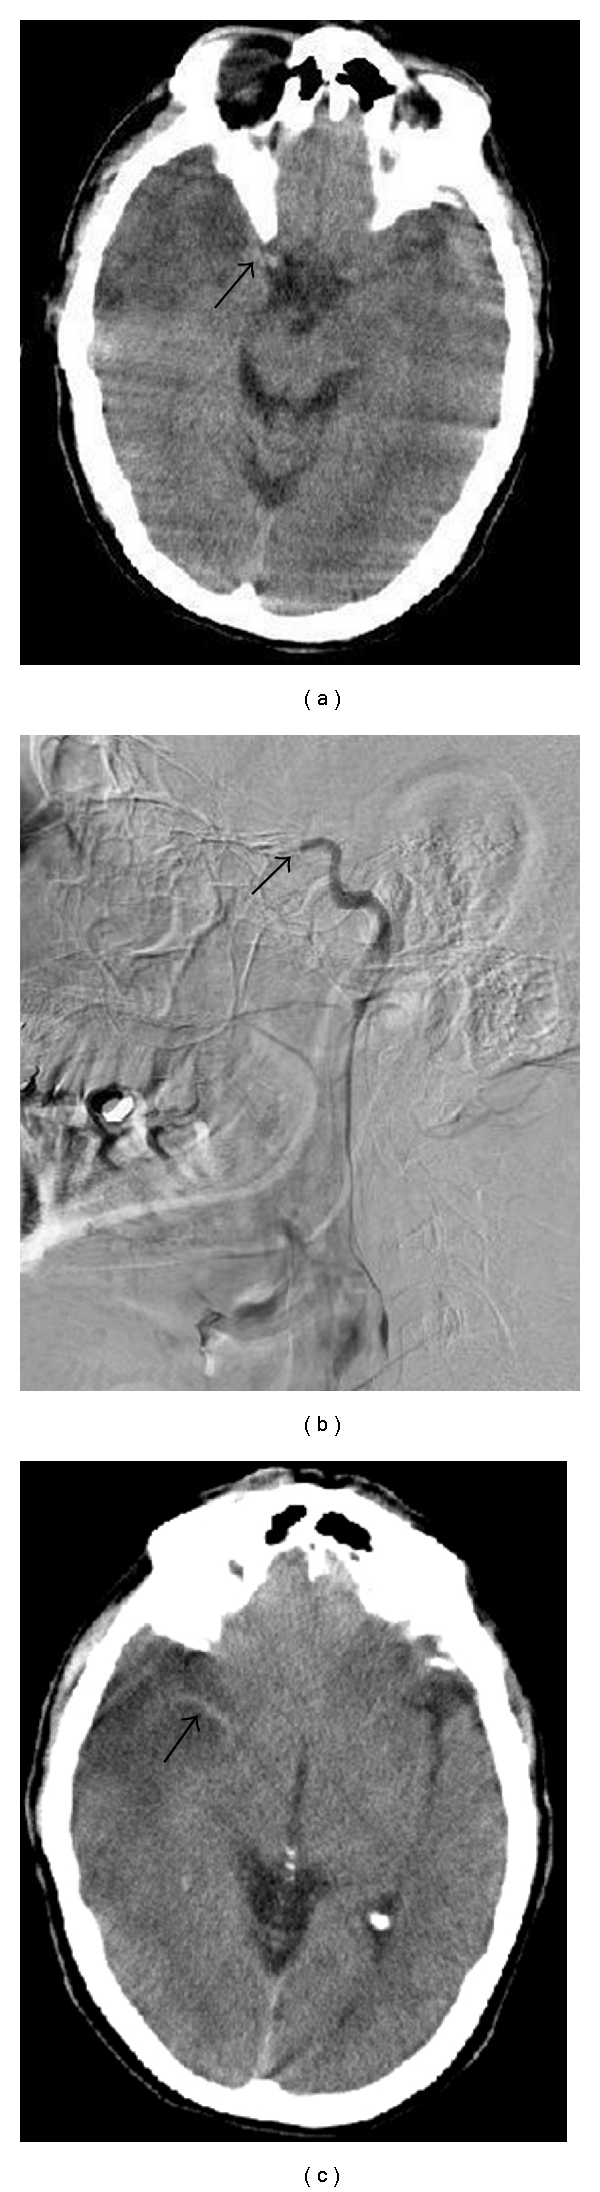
(a) NCCT demonstrating HICAS (arrow). (b) CT Carotid Angiogram demonstrating corresponding terminal carotid occlusion (arrow). (c) NCCT demonstrating HMCAS (arrow).
